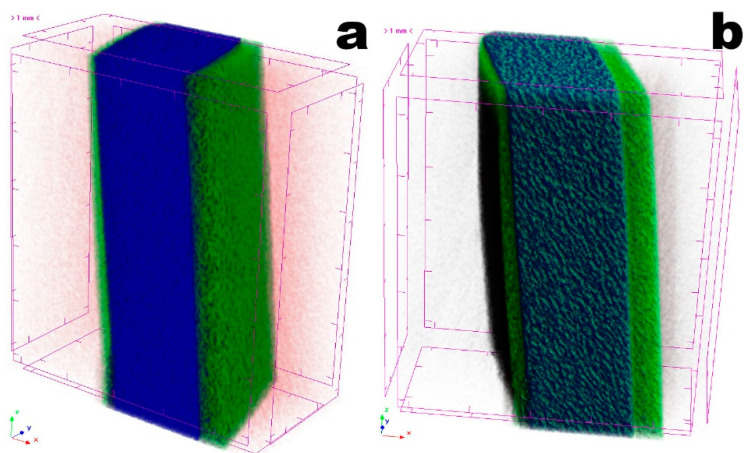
Images obtained by X-ray tomographic analyses of the bioluminescent/chromogenic hydrogel, being (a) a leaning surface view allowing us to observe the top side (right thicker surface in green), and (b) a leaning surface view allowing us to observe the bottom side (left thin surface in green).
